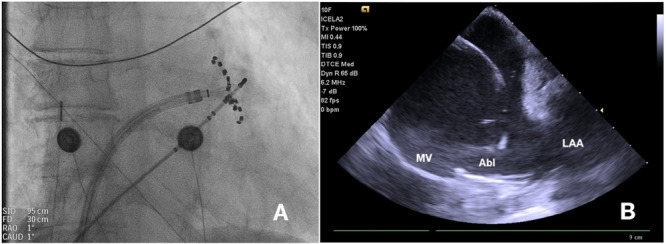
Position of the pentaspline catheter during the mitral isthmus ablation. A—Fluoroscopy antero‐posterior projection. B—Intracardiac echocardiography. Abl = ablation catheter, LAA = left atrial appendage, MV = mitral valve.
